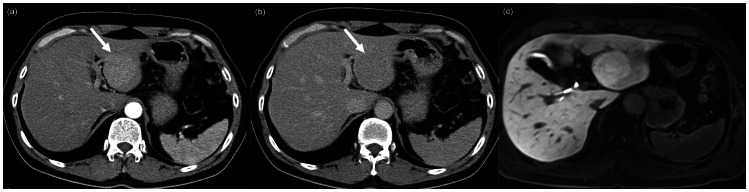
50-year-old man presented incidental liver mass. (a) On arterial phase CT image, faint arterial enhancing nodule is noted in the left lobe of the liver. (b) The lesion shows slightly low attenuation compared to adjacent liver on delayed CT image. Both reviewers diagnosed the lesion as HCC with a confidence level of 4 and 3 on CT. (c) On MR, the lesion showed high signal intensity on the hepatobiliary phase and the diagnosis was changed to FNH by both reviewers, but the lesion was confirmed as well-differentiated HCC by surgery.
